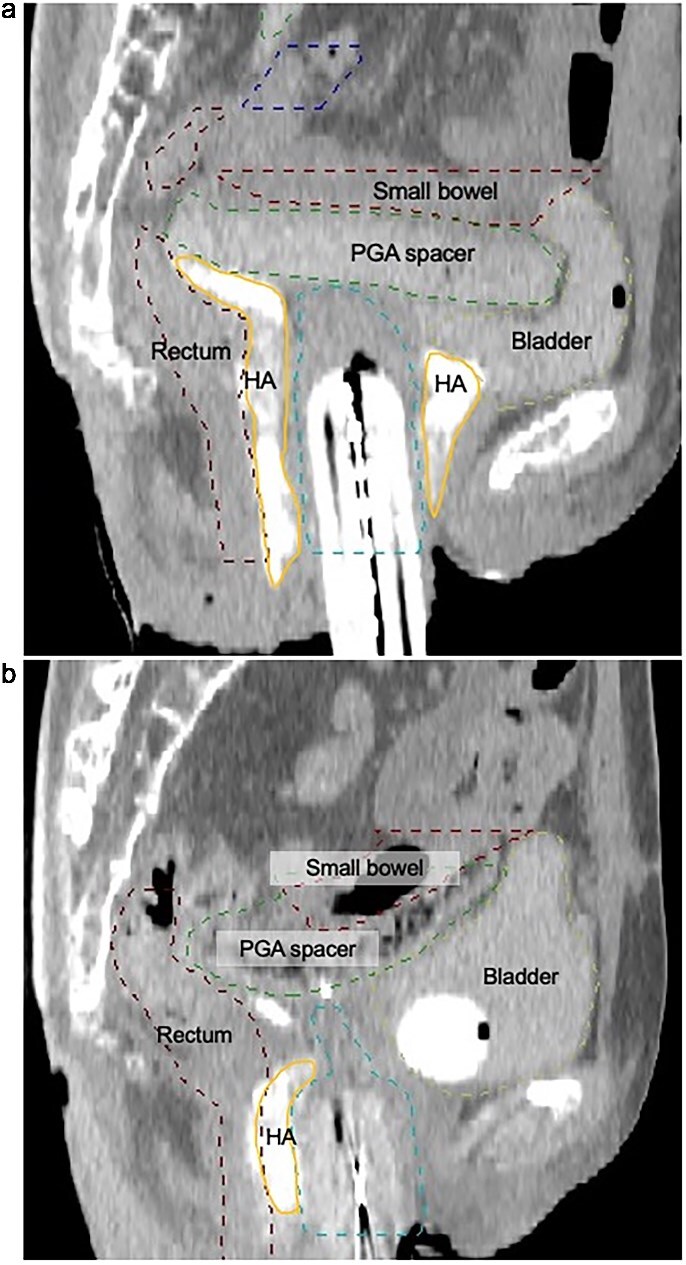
(a) Sagittal CT image acquired during the first fraction of the reirradiation HDR brachytherapy. A bioabsorbable PGA spacer (PGA spacer; green dashed contour, central) was laparoscopically placed between the recurrent tumor and the small bowel (red dashed contour, superior). Hyaluronic acid (HA) gel (HA; orange contour, bilateral anterior) was injected between the bladder and rectum to reduce dose exposure to these organs. The PGA spacer successfully created separation from the small bowel, enabling safe delivery of curative doses. The bladder and rectum are outlined with yellow (anterior) and dark red (posterior) dashed contours, respectively. (b) Sagittal CT image from the eighth fraction of HDR brachytherapy during reirradiation. The bioabsorbable spacer (PGA spacer; green dashed contour) shows partial degradation in its central region, yet maintains sufficient separation between the recurrent tumor and the small bowel (red dashed contour, superior). The spacer continues to fulfill its intended role of protecting adjacent organs. HA gel (HA; orange contour) was injected between the rectum and the applicator to reduce the rectal dose. The rectum and bladder are delineated by dark red (posterior) and yellow (anterior) dashed contours, respectively. Line styles and anatomical positions are provided alongside color for accessibility.
